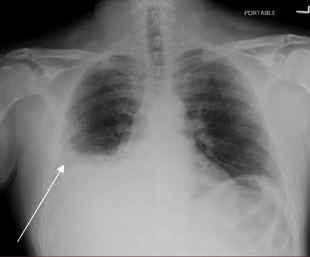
Chest radiograph. Arrow pointing at right-sided pleural effusion.
